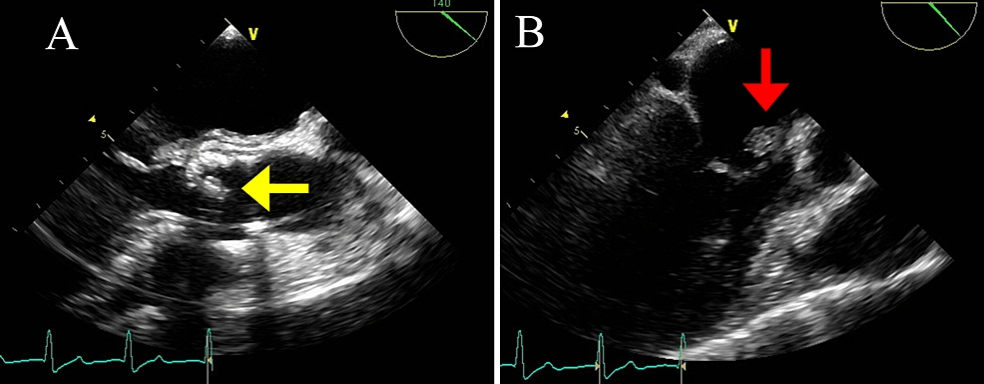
Transesophageal echocardiograms on Day 7 (A) Transesophageal echocardiogram reveals an 8-mm vegetation on the noncoronary cusp of the aortic valve (yellow arrow). (B) A 9-mm vegetation on the anterior cusp of the mitral valve was detected (red arrow).
